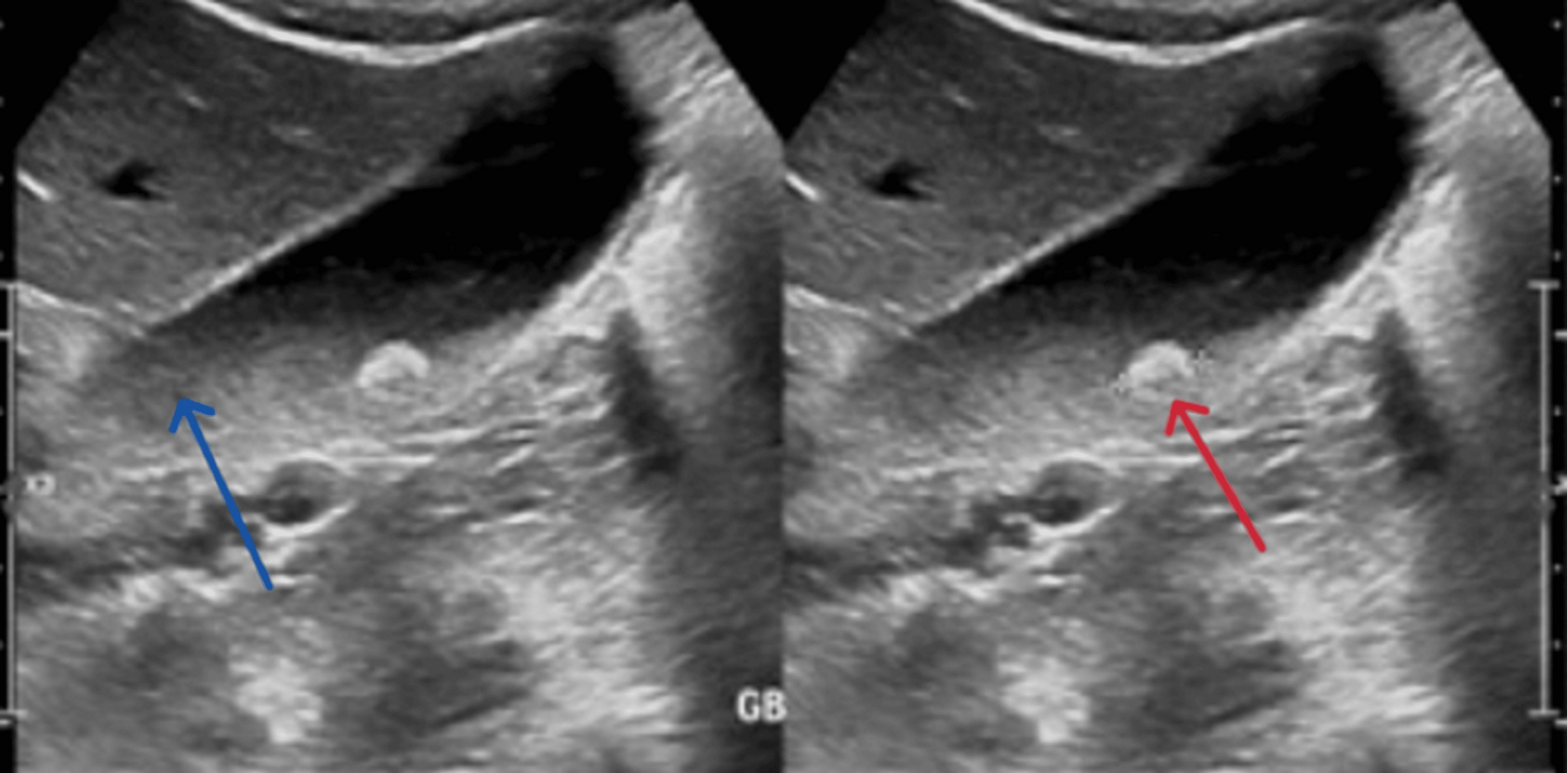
Transabdominal ultrasound findings of the gallbladder Transabdominal ultrasound demonstrates a distended gallbladder with an 8 mm gallstone (red arrow) and associated thick sludge formation (blue arrow). Mild gallbladder wall thickening is also noted, consistent with acute calculous cholecystitis.
